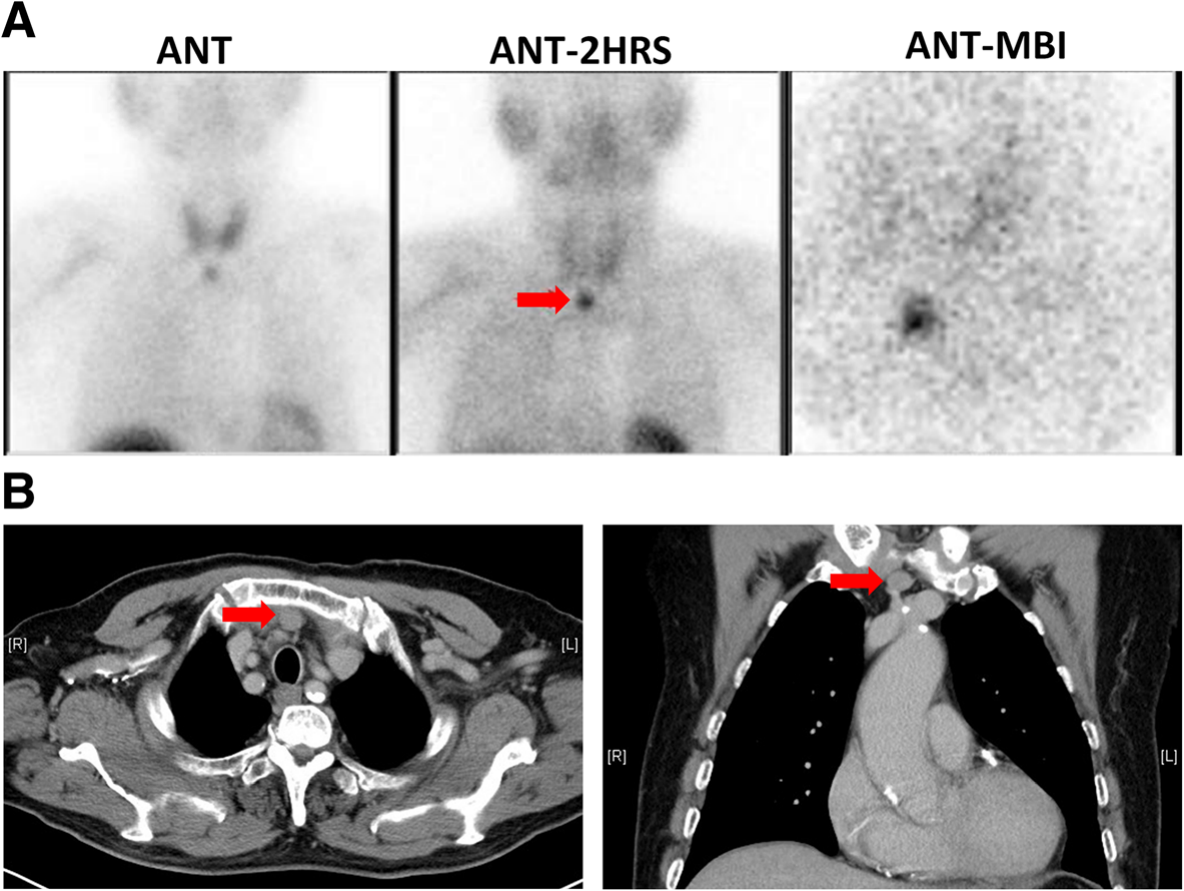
a Parathyroid scan with Tc-99 m MIBI, (b) Post contrast chest and mediastinal CT scan. The arrow indicates the location of the ectopic parathyroid
